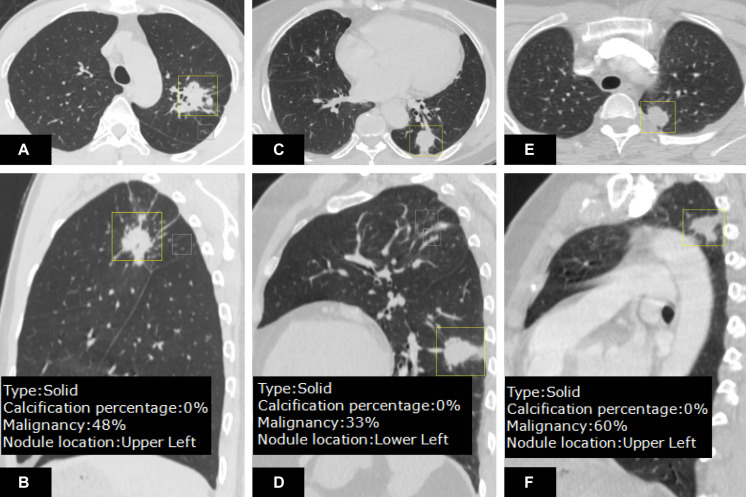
On the CT images (A,B), an irregular nodule with malignancy <50% rated by DL-CADx was diagnosed as malignant by double reading (malignant likelihood: 75–100%), which turns out to be granulomatous nodule in pathology. For the nodule detected on the CT images (C,D), DL-CADx considered it more likely to be a benign nodule, but double reading classified it as a malignant nodule (malignant likelihood: 75–100%), which was confirmed as adenocarcinoma. For the pathologically confirmed granuloma on the images (E,F), 60% of malignancy risk was rated by DL-CADx, but double reading considered it more likely to be benign (malignant likelihood: 25–50%).
